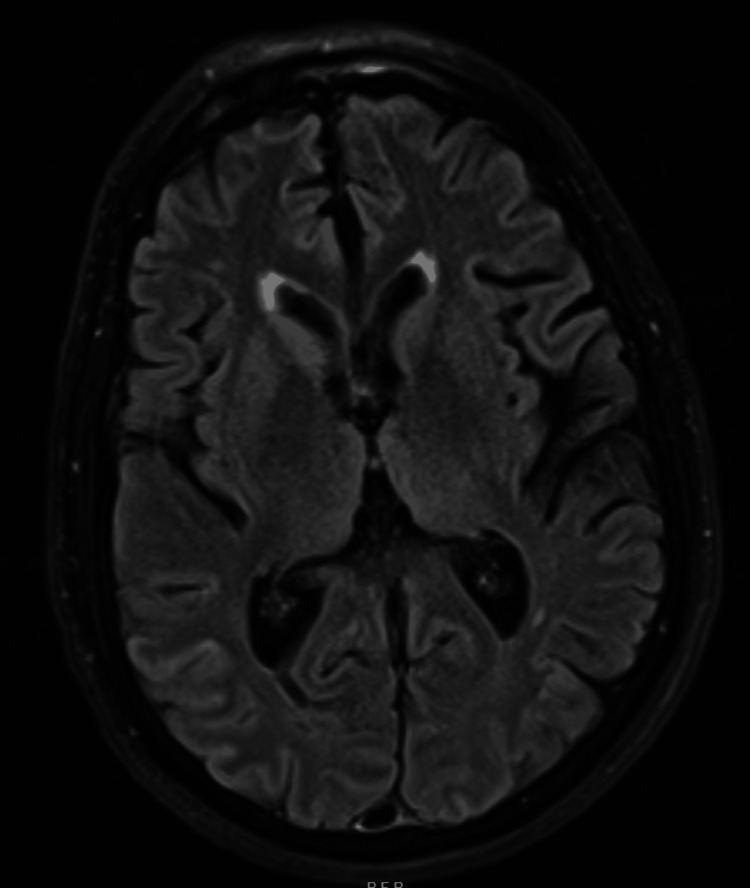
MRI T2 FLAIR showing hyperintensity in bilateral caudate nuclei, right putamen FLAIR: fluid-attenuated inversion recovery
